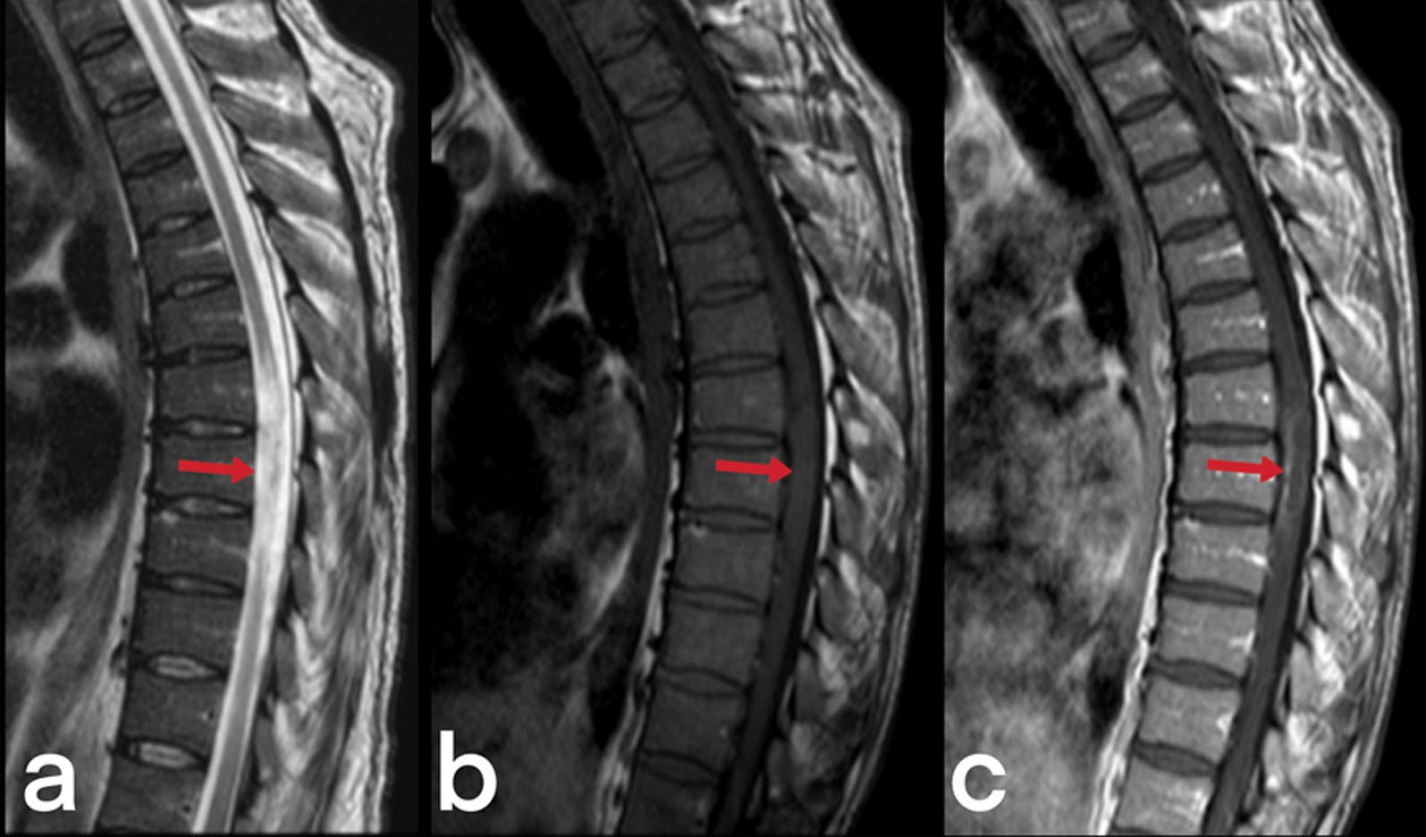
MR images of a 56-year-old male with the diagnosis of tuberculous myelitis. Sagittal T2 weighted image (a) shows a heterogeneous increased signal on the spinal cord (arrow). Pre-contrast (b) and post-contrast (c) T1 weighted images reveal linear contrast enhancement of the same region (arrows)
